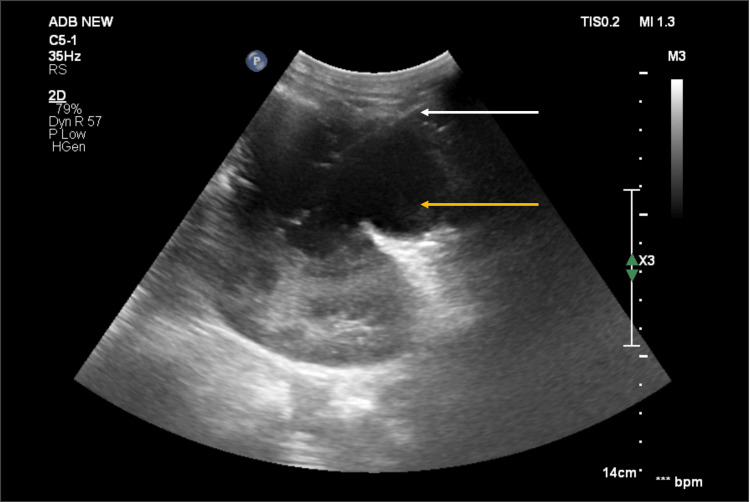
Ultrasonographic image of the renal abscess. The yellow arrow indicates the renal and perirenal collection measuring 10.6 x 6.3 cm. The white arrow points to the pigtail catheter in the abscess cavity.
